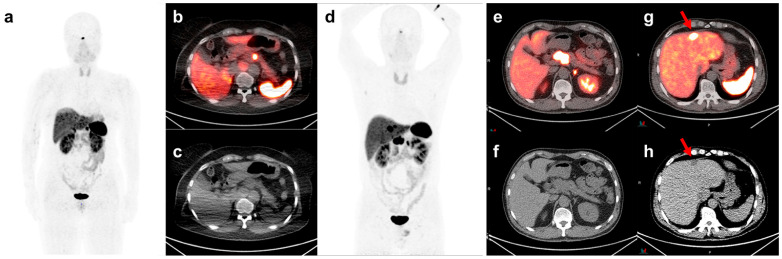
[^68^Ga]-DOTATOC PET/CT maximum intensity projection (MIP), axial fusion, and CT images of: (a–c) A 60-year-old woman with a G2 pNET located in the pancreatic body and (d–h) A 51-year-old man diagnosed with G2 pNET. The primary tumor in the pancreatic head is noted (e,f). Hepatic metastasis was observed on the axial fusion and CT images ((g,h) red arrows). The metabolic volume of the primary tumor was higher in the patient with initial hepatic metastasis than in the patient without metastasis (TLSRE: 266.96 vs. 16.07, SRETV: 7.23 vs. 0.94). The values of other PET/CT indices were as follows: SUVmax: 65.37 vs. 31.72, SUVmean: 36.91 vs. 17.08. Abbreviations: Ga, Gallium; PET/CT, positron emission tomography/computed tomography; SUV, standardized uptake value; max, maximal; SRETV, somatostatin receptor-expressing tumor volume; TLSRE, total lesion somatostatin receptor expression.
